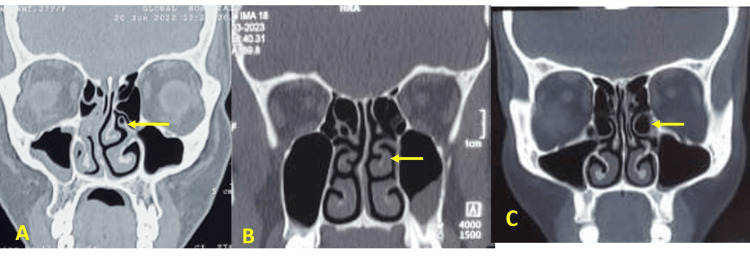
Coronal CT images of paranasal sinus showing other variations A: pneumatized uncinate process; B: bilateral paradoxical middle turbinate; C: left hypertrophied ethmoidal bulla CT: computed tomography
